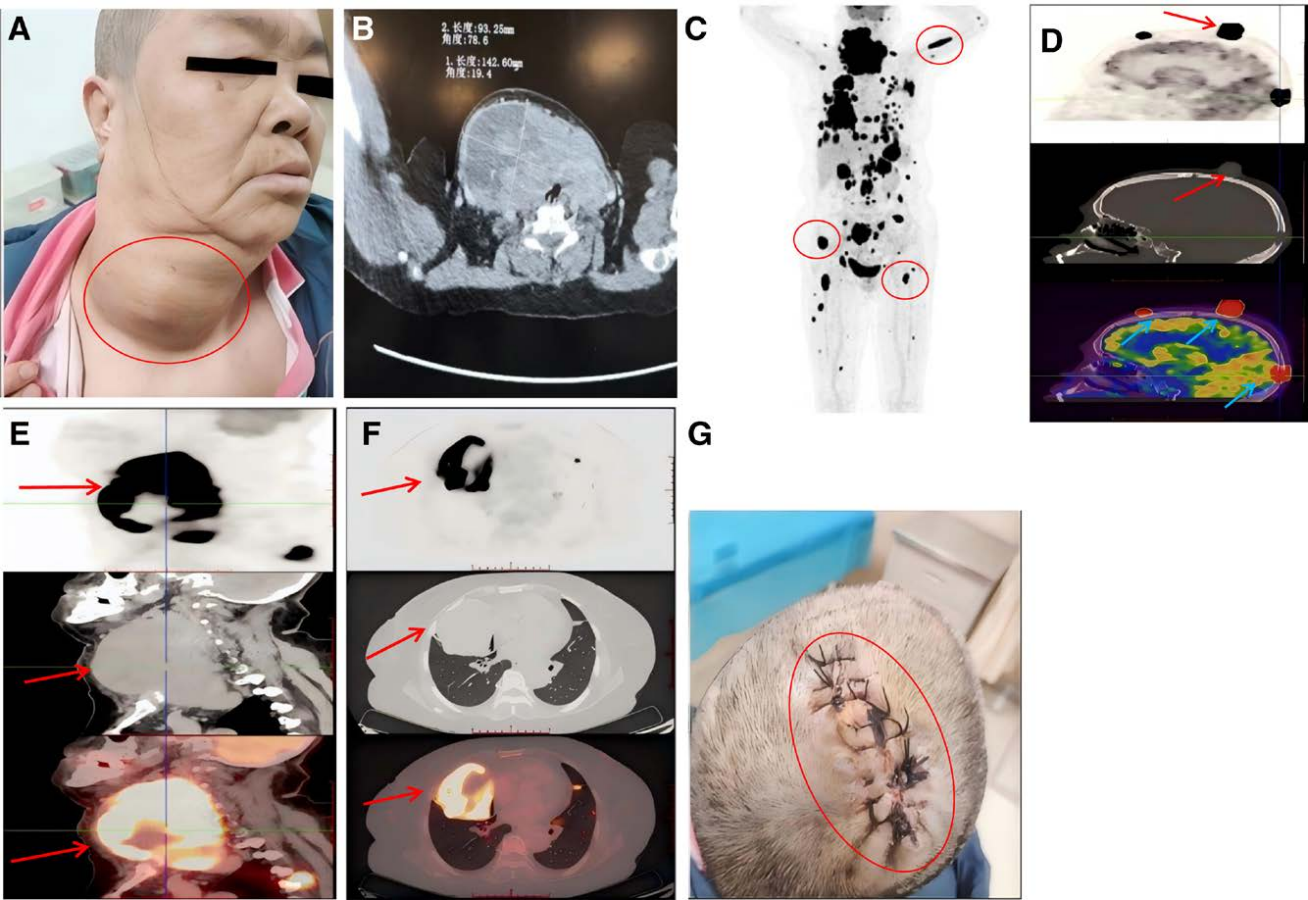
(A) Thyroid lesion. (B) CT measurement of thyroid lesions. (C) Whole-body bone scintigraphy multiple bone metastases. (D) PET CT imaging of scalp metastases. (E) PET-CT imaging of thyroid lesions. (F) PET CT imaging of lung metastatic lesions. (G) After scalp mass excision. CT = computed tomography, PET-CT = positron emission tomography-computed tomography.
